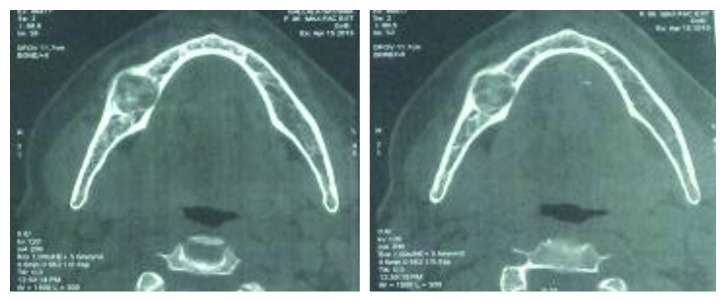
Computed tomography scan: axial views showing multiloculated ground-glass ossification of the lesion.
